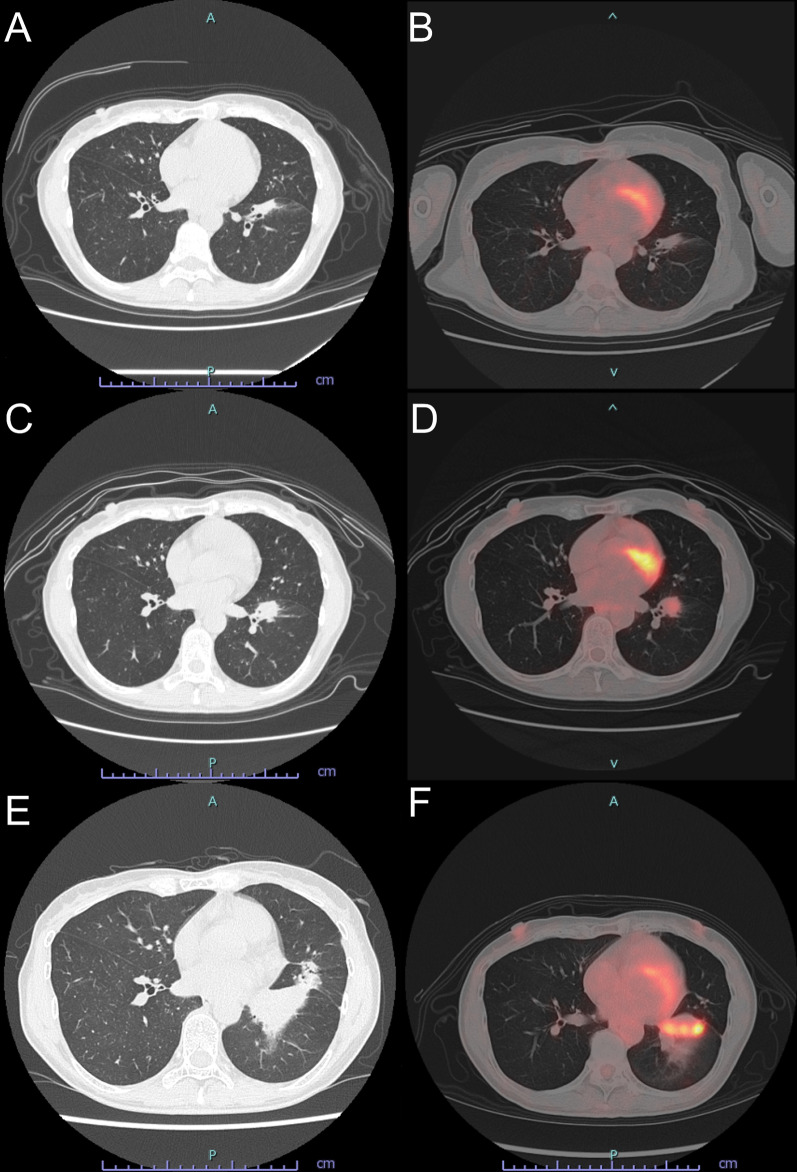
At 6 months after osimertinib administration, A CT imaging showed shrinkage of the primary tumor and B PET-CT findings indicated a low SUVmax level of 1.5. After 1.5 years, C the primary tumor showed regrowth and D SUVmax was increased to 4.55, thus SRT was performed. At 3.5 years after SRT, E the primary tumor showed continued growth and F SUVmax was increased to 16.78, which led to reference to our hospital
